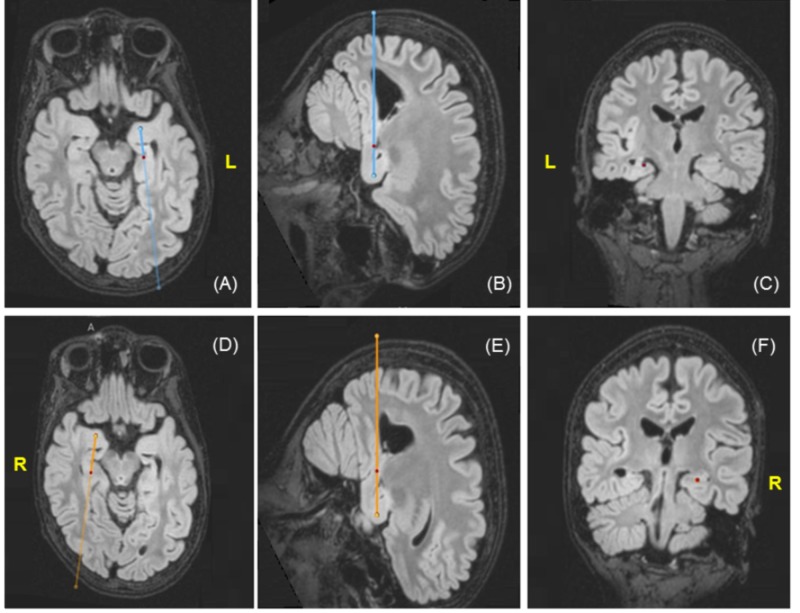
Stealth magnetic resonance images demonstrating the planned trajectory for the left-sided (A-C) and right-sided (D-F) leads The red circles represent the hippocampal target (D-F).
